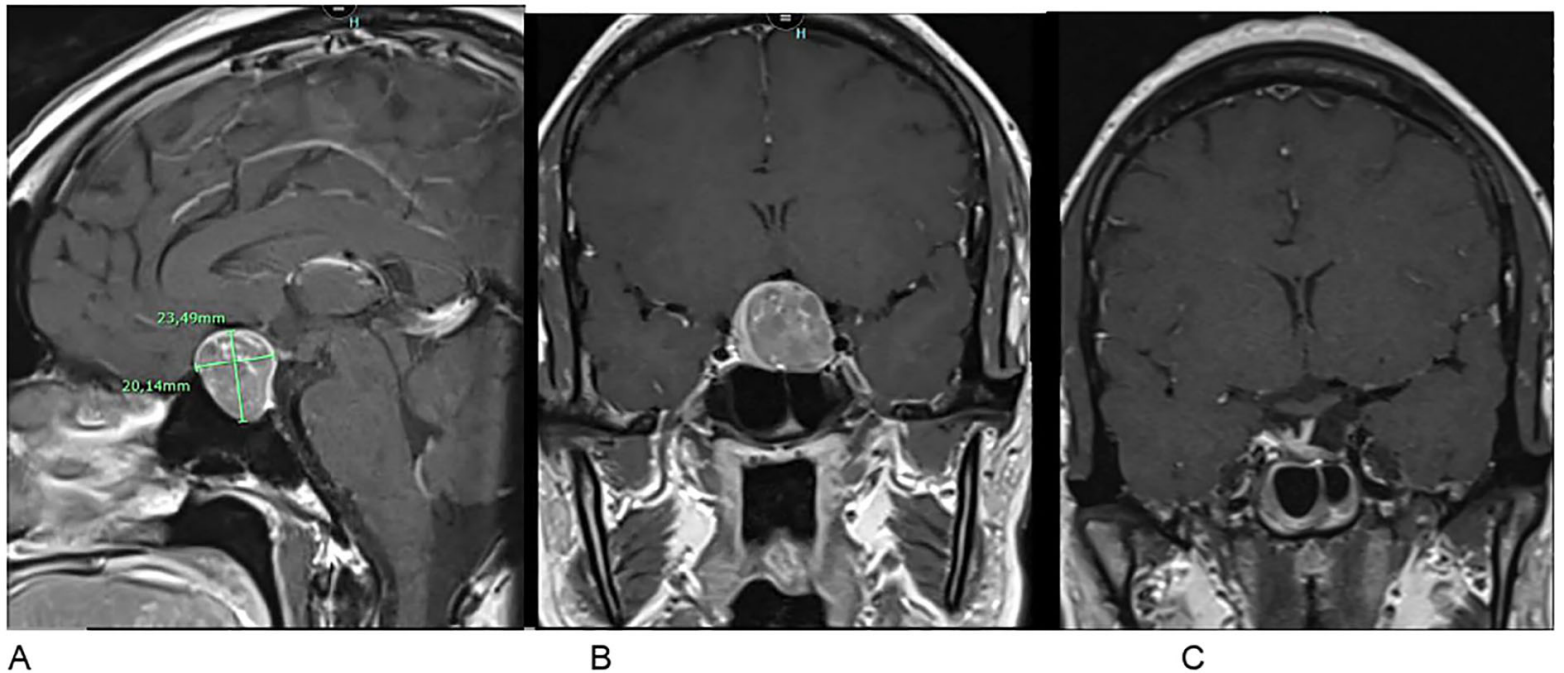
Pituitary MRI in T1-weighted post-contrast sequences. (A, B) Preoperative frontal and sagittal views showing a pituitary macroadenoma (23.5 × 20.1 mm) with optic chiasm involvement. (C) Postoperative frontal view at 3 months showing regression of the tumor mass.
